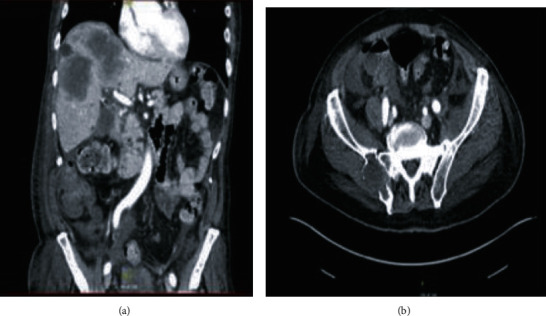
CT of the abdomen and pelvis with IV contrast demonstrating hepatic metastatic disease with an enlargement of the hepatic lobe mass. Right adrenal mass consistent with metastatic disease. Development of portacaval adenopathy consistent with metastatic disease (a). Expansile osteolytic right posterior ilium lesion (b).
